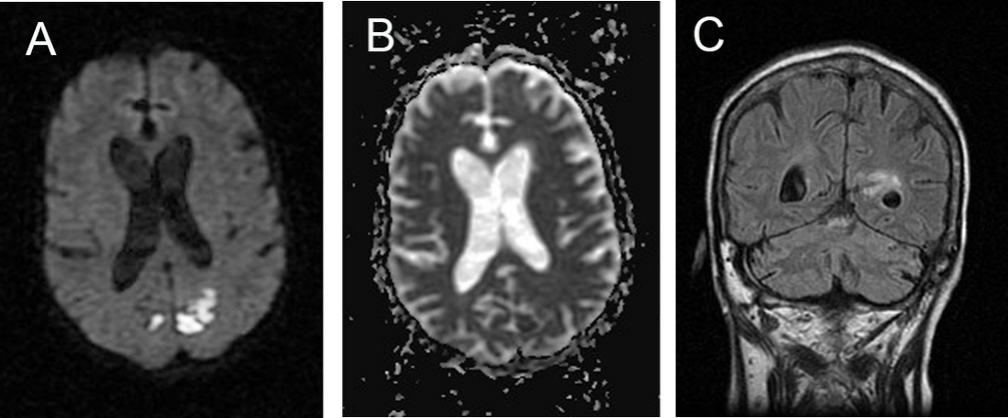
MRI in patient with cerebral lymphoma. Note patchy restricted diffusion in the posterior cortex (A) with partial confirmation on ADC (B) together with abnormal signal in the periventricular area and white matter on FLAIR (C). These areas corresponded to leptomeningeal nodular enhancing soft tissue. ADC, apparent diffusion coefficient.
